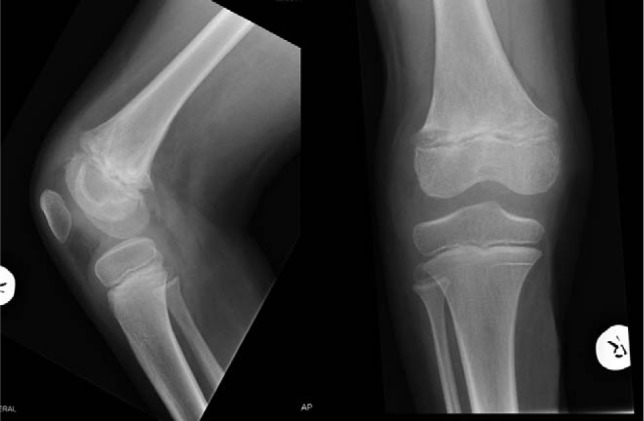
AP and lateral radiographs at 1 year showing no skeletal abnormality.
